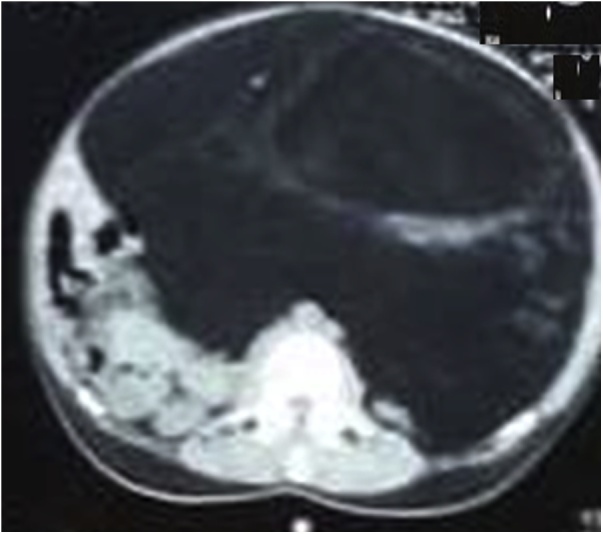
Coronal computed tomography scan showing fat density mass occupying all abdominal cavity.
